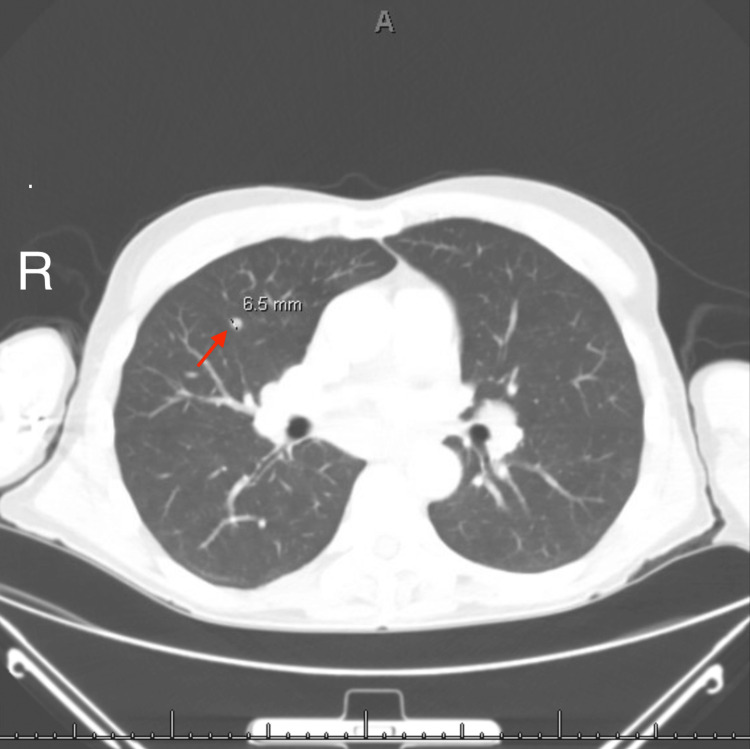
Chest CT depicting an intrapulmonary lymph node measuring 6.5 mm. CT: computed tomography.
